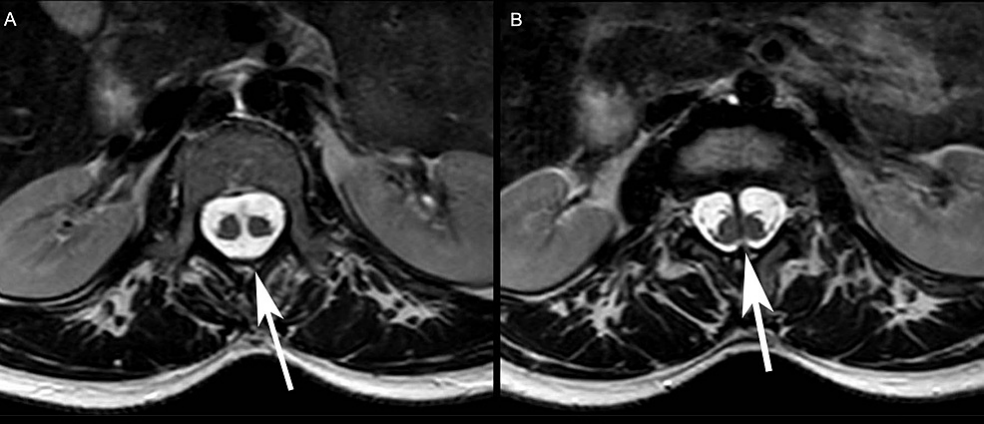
A: T2-weighted image, axial plane, level of L2, shows a single dural sac containing both hemicords with appearance of the chondro-osseous ligament central in anteroposterior orientation. B: T2-weighted image, axial plane, level of L2–L3, shows two hemicords separated by the chondro-osseous ligament, each in a separate dural sac.
